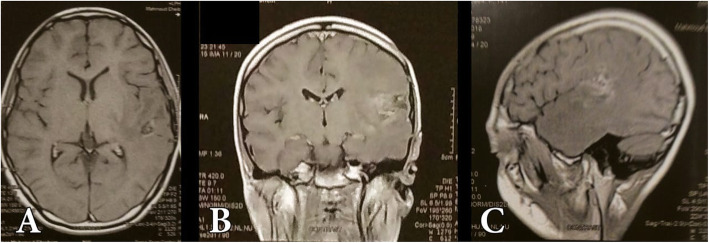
MRI with gadolinium follow-up of the same patient showing total resection of the tumor, pathology revealed astrocytoma grade III
